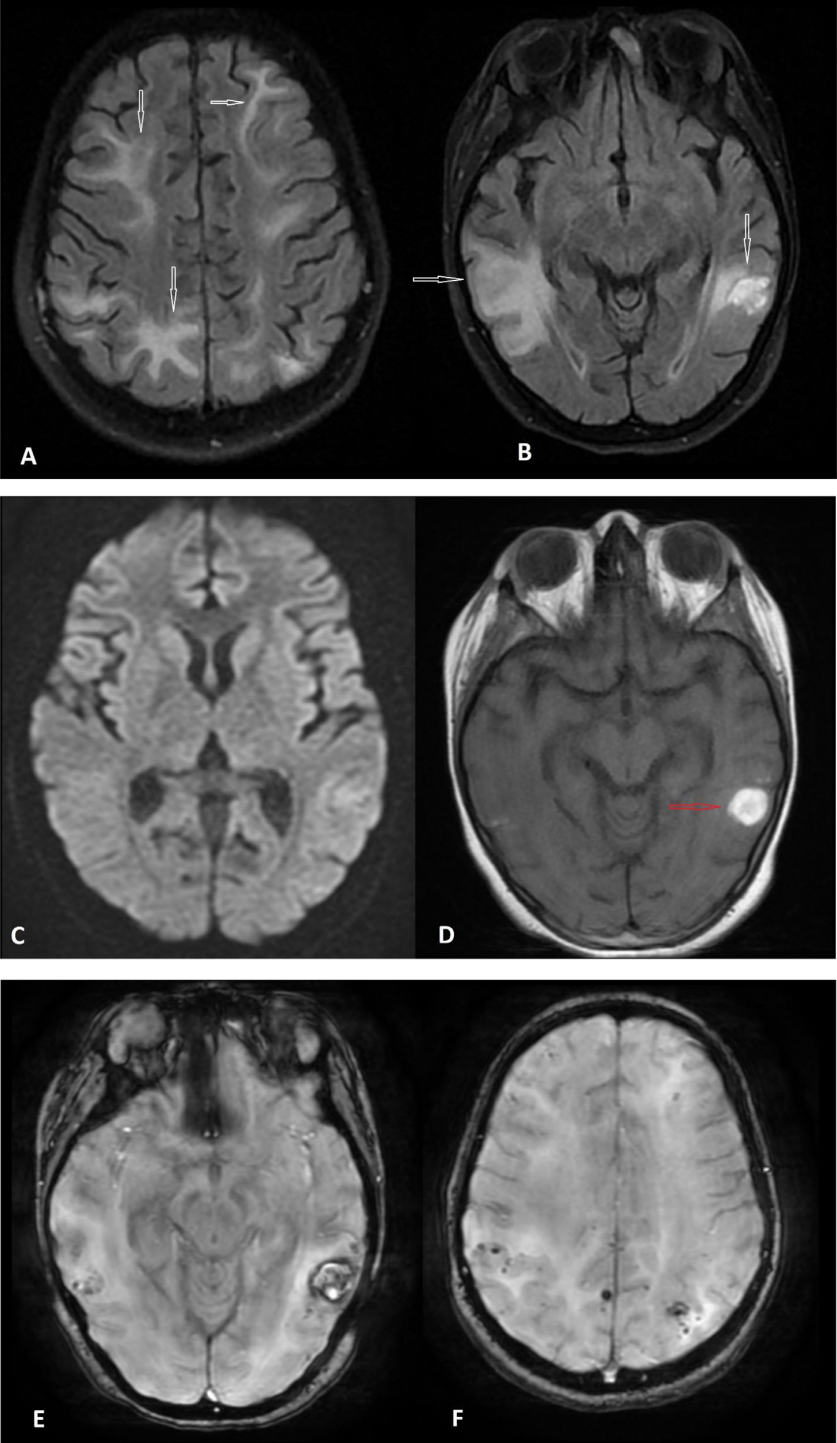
Axial plane of the brain magnetic resonance imaging without gadolinium T2/FLAIR (A, B) sequences: there are diffuse hyper signal intensities in both cerebellar hemispheres at the gray-white matter junction of the parietooccipital, frontal, and temporal lobes (white arrows). But no diffusion restriction exists on the DWI sequence (C). Hypersignal foci on T1W sequence (D) are seen (red arrow), representing hemorrhage which shows low signal intensity on SWI sequences (E, F).
